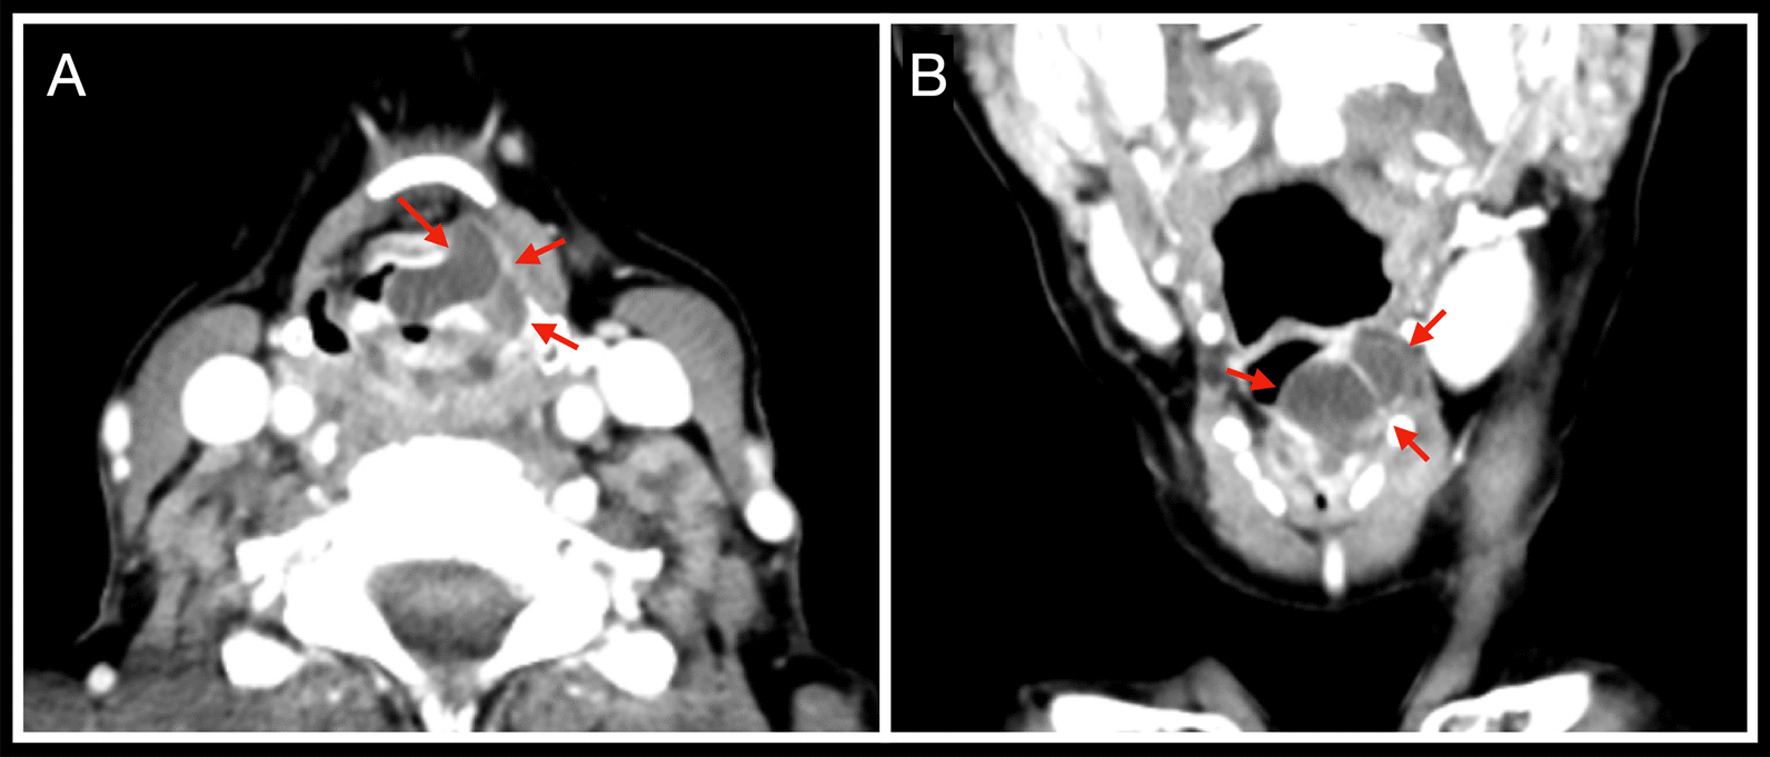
Head and neck CT showing a bilobed cystic neoformation rising from the left Morgagni ventricle. (A) Axial acquisition. (B) Coronal acquisition. The red arrows outline the borders of the lesion
